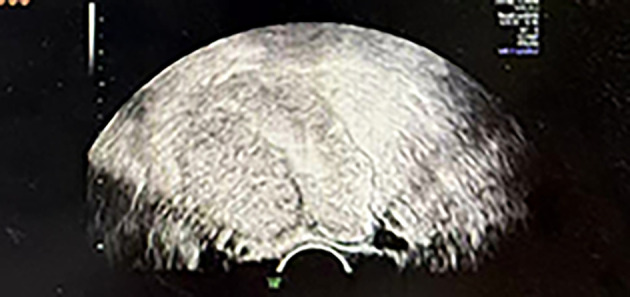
Obstetric ultrasound examination. Ultrasonography revealed a solid pelvic mass measuring approximately 6.8 × 6.3 cm located posterior to the gravid uterus.
